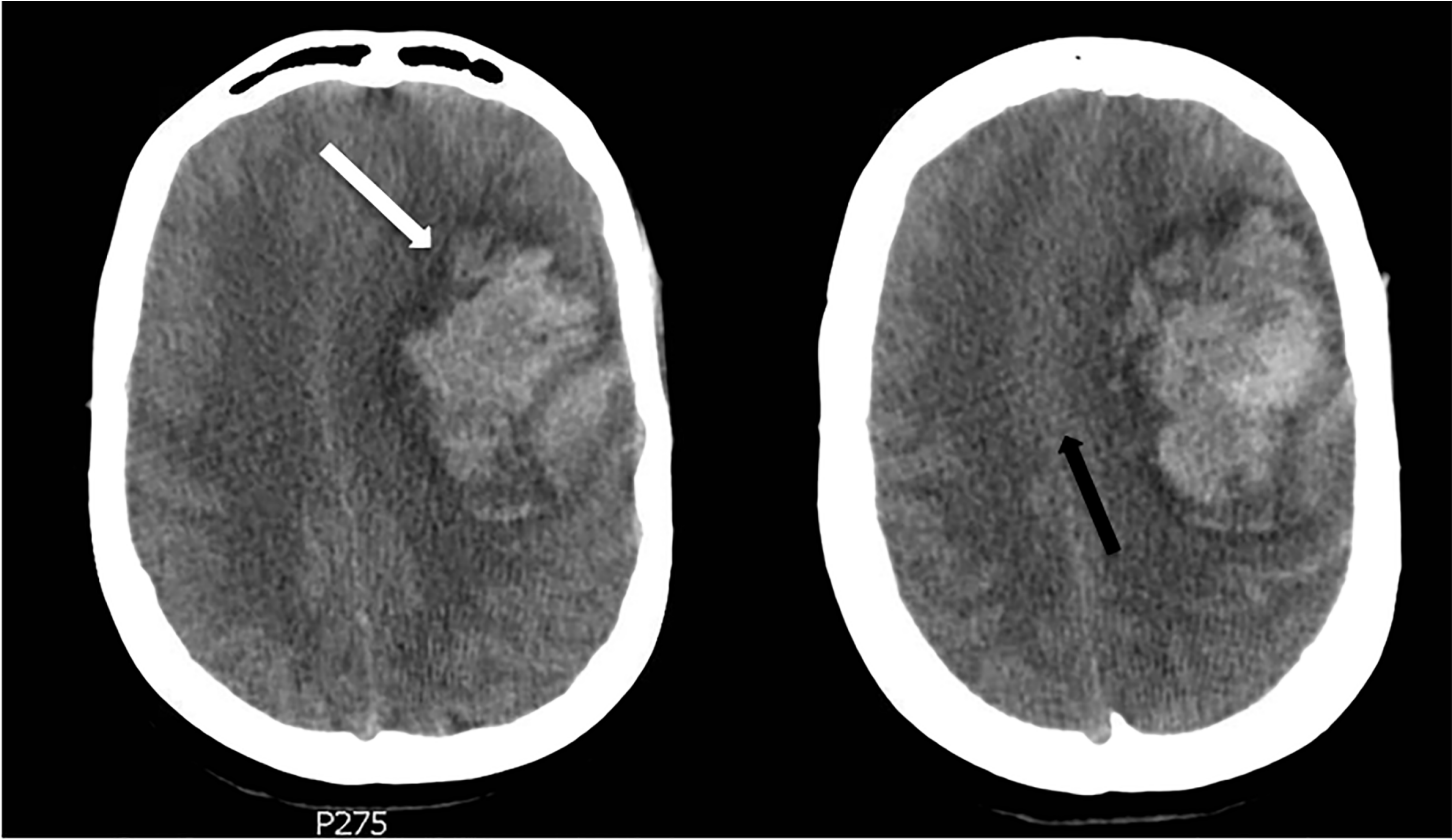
Computed tomography of the brain, axial images, demonstrating 7.2 centimeter parenchymal hematoma (white arrow) with associated edema causing 10 millimeters of midline shift (black arrow).
